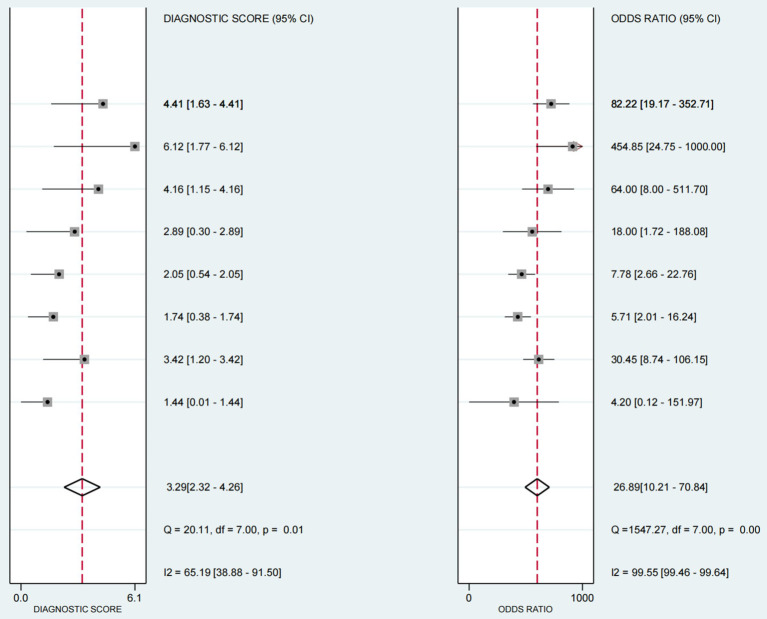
Forest plot of diagnostic scores and odds ratios in ultrasound-based dysphagia assessment (with 95% CI). Diagnostic scores and ratios of each individual study in the assessment of dysphagia by ultrasound. The diagnostic score and odds ratio, each of which provides a 95% Confidence Interval (CI), as well as the results of several statistical tests, such as the Q statistic and the I^2^ statistic.
